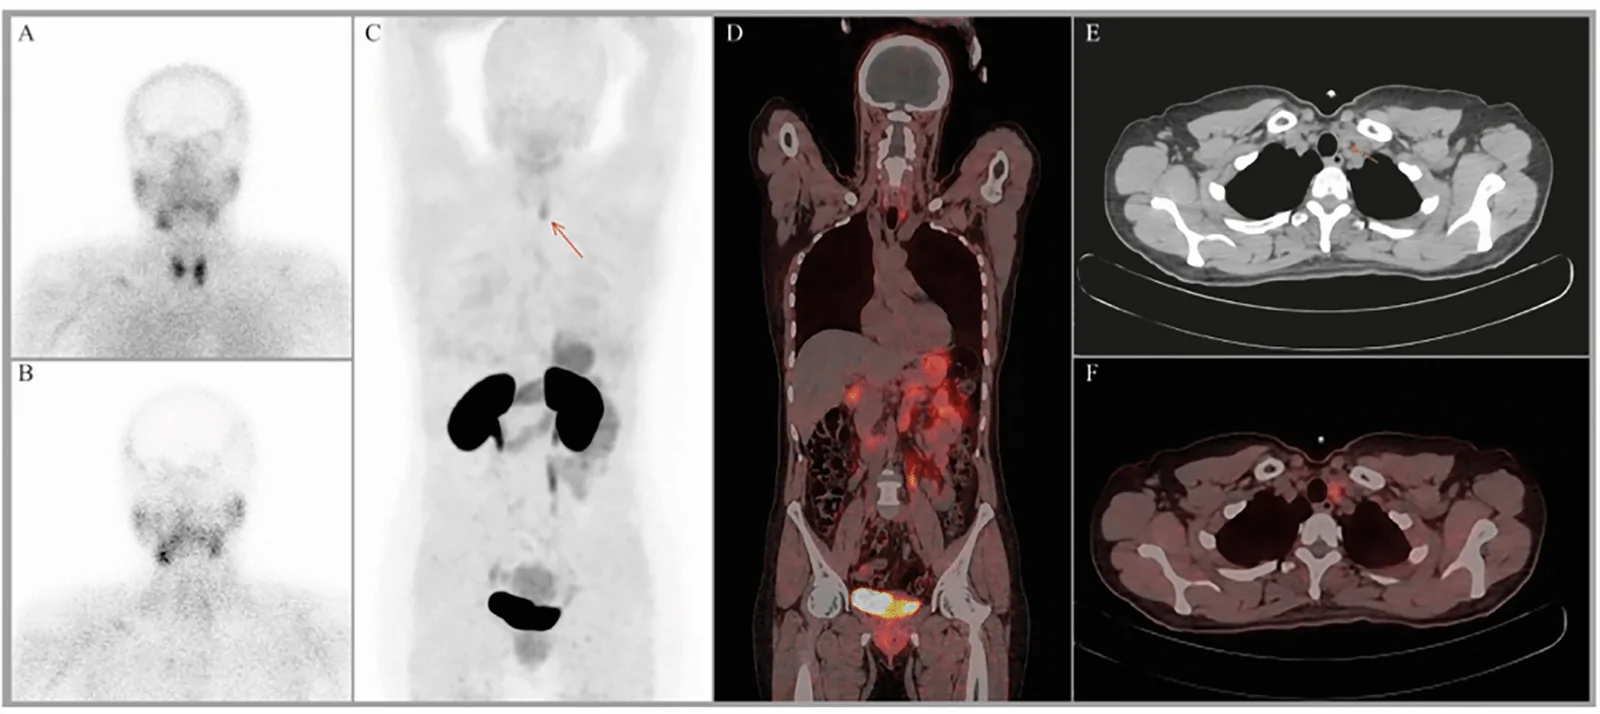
Parathyroid adenoma detected on ⁶⁸Ga-Trivehexin PET/CT in the absence of typical scintigraphic findings. (A) Early-phase ⁹⁹ᵐTc-sestamibi SPECT gamma-camera image demonstrating physiological thyroid uptake without abnormal focal uptake suggestive of parathyroid adenoma. (B) Delayed- phase ⁹⁹ᵐTc-sestamibi image showing no persistent focal uptake suggestive of parathyroid adenoma. (C) Maximum intensity projection (MIP) image of ⁶⁸Ga-Trivehexin PET/CT revealing a focal tracer uptake in the left cervical region. (D) Fused PET/CT coronal image showing focal radiotracer uptake corresponding to the left-sided lesion. (E) Corresponding axial CT image at the same anatomical level. (F) Fused PET/CT axial image confirming focal tracer uptake with precise anatomical localization
